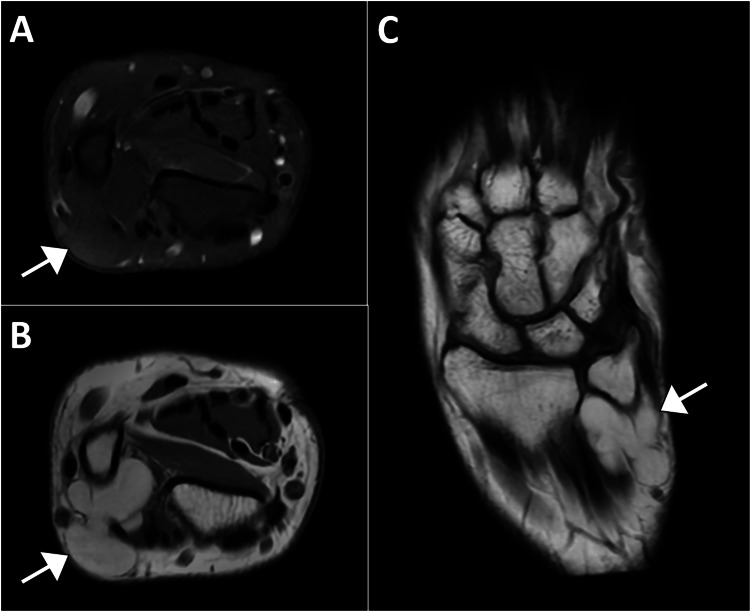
Magnetic resonance imaging of the left wrist (Panel A displays an axial proton density short tau inversion recovery image, Panel B shows an axial T1-weighted image without fat saturation, and Panel C shows a coronal proton density image) showing a fat-containing mass (arrow) within the extensor indices muscle. The mass adheres to the posterior wall of the tendon, pierces the muscle fascia, and extends into the subcutaneous tissue, giving it a bilobed appearance. It measures 2.2 cm x 2 cm and extends 2.2 cm along the longitudinal axis. The lesion is near the neurovascular bundle and abuts the radio-ulnar interosseous membrane, without intra-articular extension.
